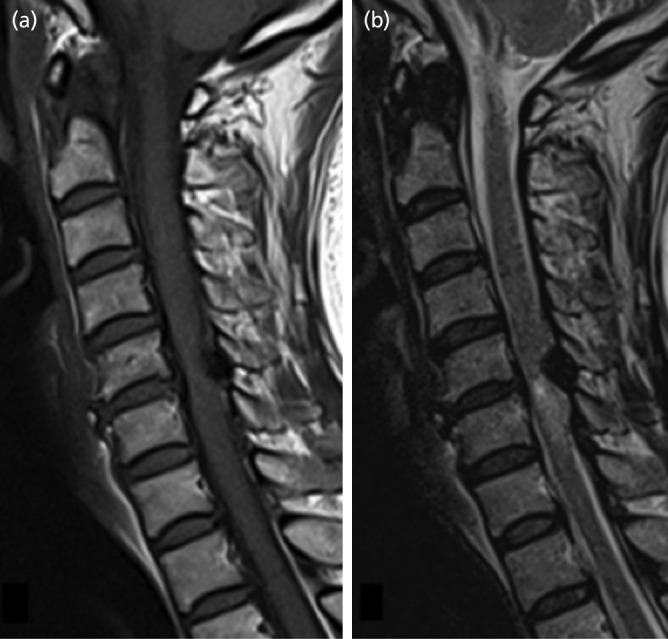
Case 4. MR imaging demonstrating osseous lesion protruding into the spinal on the abdominal side of the lamina of the C5. (a) sagittal T1-weighted image, (b) sagittal T2-weighted image.
